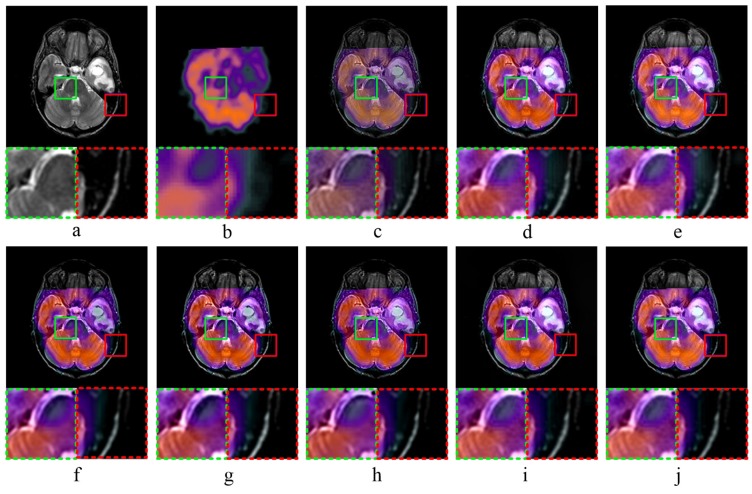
MR-SPECT image fusion Experiments 2: (a,b) source images; and (c–j) the fused image obtained by MST-SR, NSCT-PC, NSST-PCNN, ASR, CT, KIM, CNN-LIU, and the proposed method, respectively. Two partially enlarged images marked in green and red dashed frames correspond to the regions surrounded by green and red frames in the fused image.
